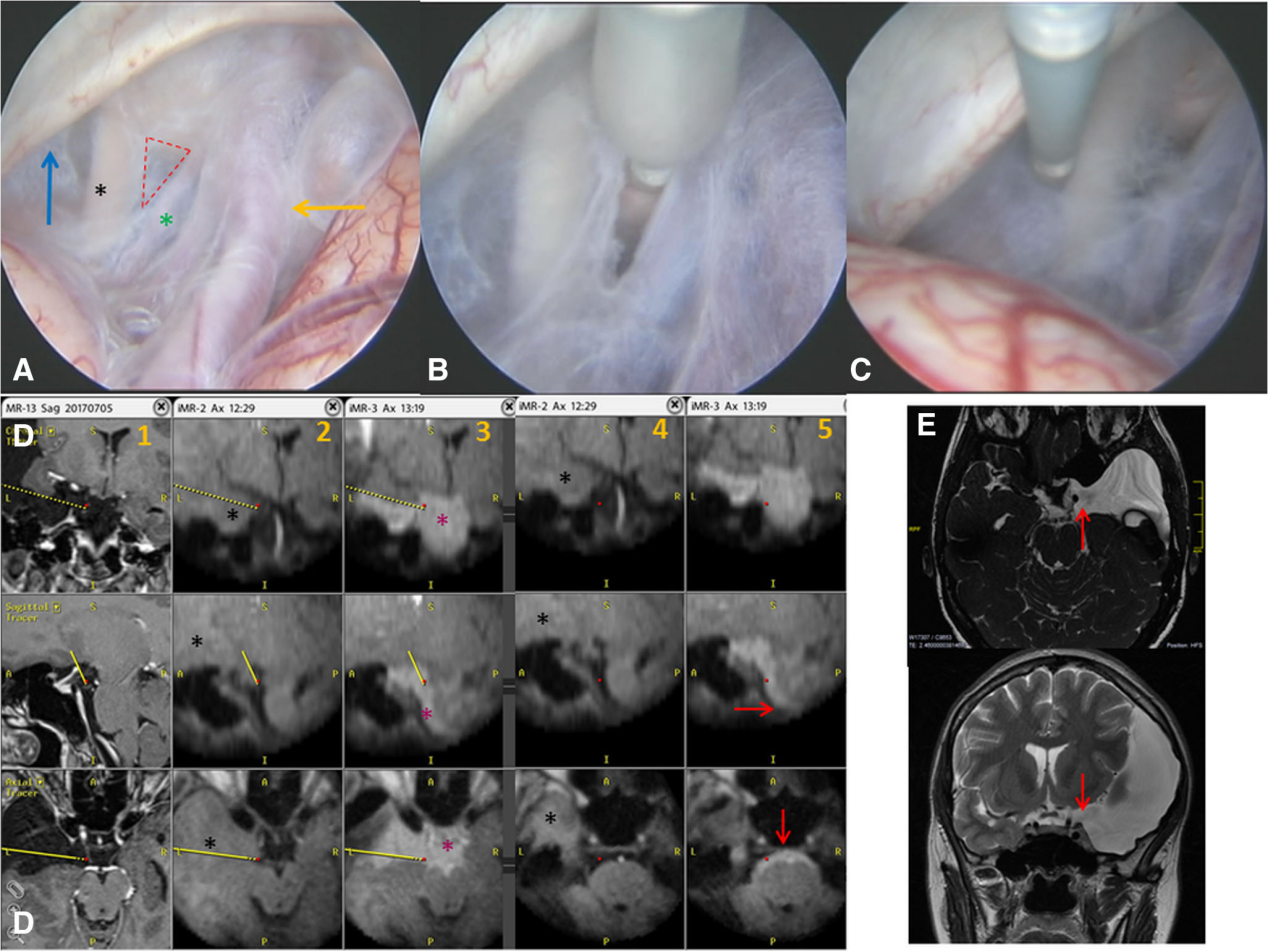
Images showing the endoscopic cystocysternostomy performed in a 35-year-old woman (patient 19) suffering from a large middle fossa arachnoid cyst. a Endoscopic view from the inside of the cyst. The red dotted lines are pointing the triangular area of planned fenestration, between the left oculomotor nerve (black asterisk) and the posterior communicating artery (green asterisk). The yellow arrow is showing the internal carotid artery while the blue one the anterior petroclinoidal fold. b The cyst fenestration is enlarged with a 3F Fogarthy catheter. c The catheter is exploring the area between the oculomotor nerve and the petroclinoidal fold for a possible second cyst fenestration. Yet based on the positive results from iMR cysternography that showed an effective contrast flow through the first fenestration (D), we resigned from performing a second one. d Radiological view of the arachnoid cyst before its fenestration (column 1—high-field 1.5-T images, columns 2 and 4—low-field intraoperative 0.15-T images after contrast administration into the cyst; the area containing the diluted gadolinium contrast is pointed with a black asterisk) and after the cystocysternostomy (columns 3 and 5). The yellow line is showing the surgical plan for cyst fenestration. Prefenestration intraoperative MR cysternography demonstrated no contrast flow from the cyst to the neighboring cysterns, while the postfenestration cysternography confirmed clearly an efficient contrast flow not only to the arachnoid cysts localized close to the fenestration point (column 3, pink asterisk) but also in more distally located cysts (column 5, red arrow). e Postoperative axial heavily T2-weighted steady-state image (3D-DRIVE), and T2-weighted coronal 3-T MRI scans performed 6 months after surgery. Red arrows point the side of cyst fenestration with visible flow void effect. We observed additionally a decrease of the cyst volume from 127.3 to 115.3 cm^3^ and a decrease of the midline shift from 4.5 to 3 mm
